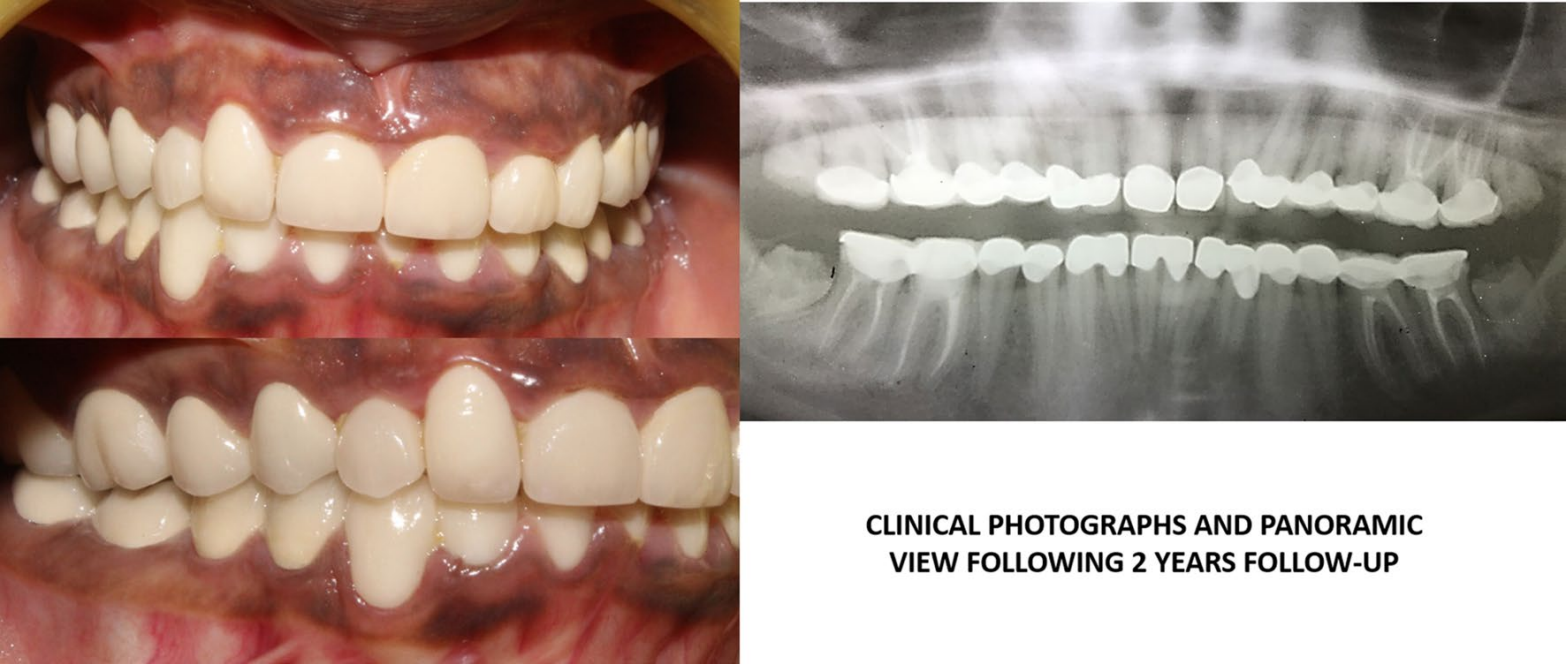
Postoperative follow-up clinical photographs and orthopantomogram view after 2 years
